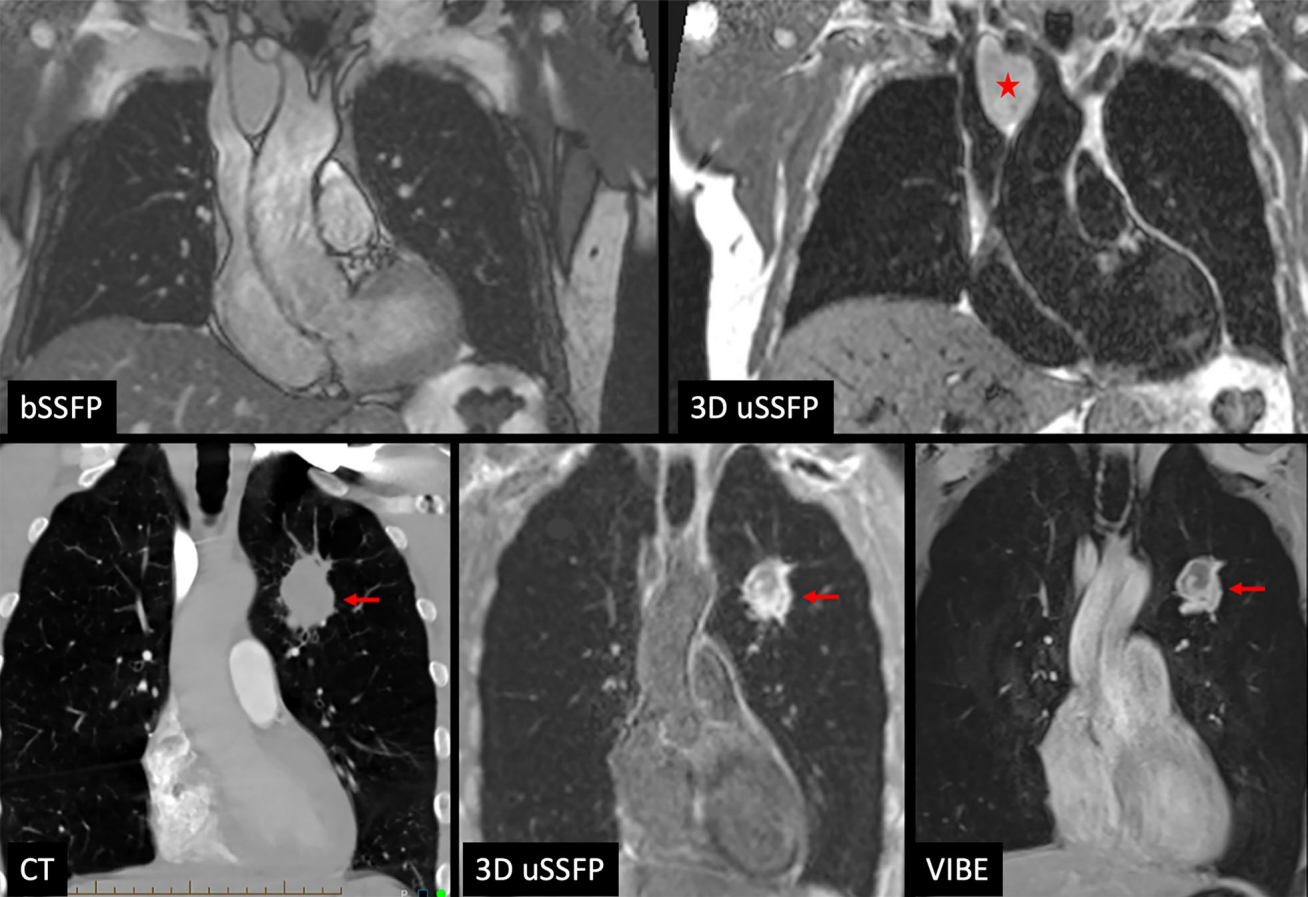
Breath-hold 3D uSSFP in two different patients. *Top row* Non-contrast images in a patient with a benign mediastinal cyst (*). Left: 2D bSSFP; right 3D uSSFP. In the 2D bSSFP image, the cyst is difficult to distinguish from the adjacent, nearly isointense brachiocephalic vein, aortic arch, and branch vessels, but is easily visualized with 3D uSSFP due to the low blood pool signals. *Bottom row* Postcontrast images in a patient with a necrotic, biopsy-proven non-small cell lung cancer (red arrows). Left: CT; middle: 3D uSSFP; right: VIBE. Since the 3D uSSFP images were obtained after contrast administration, the blood pool appears gray rather than black. Nonetheless, 3D uSSFP better demonstrates the tumor spiculations and provides higher contrast between the tumor and cardiovascular structures than VIBE
